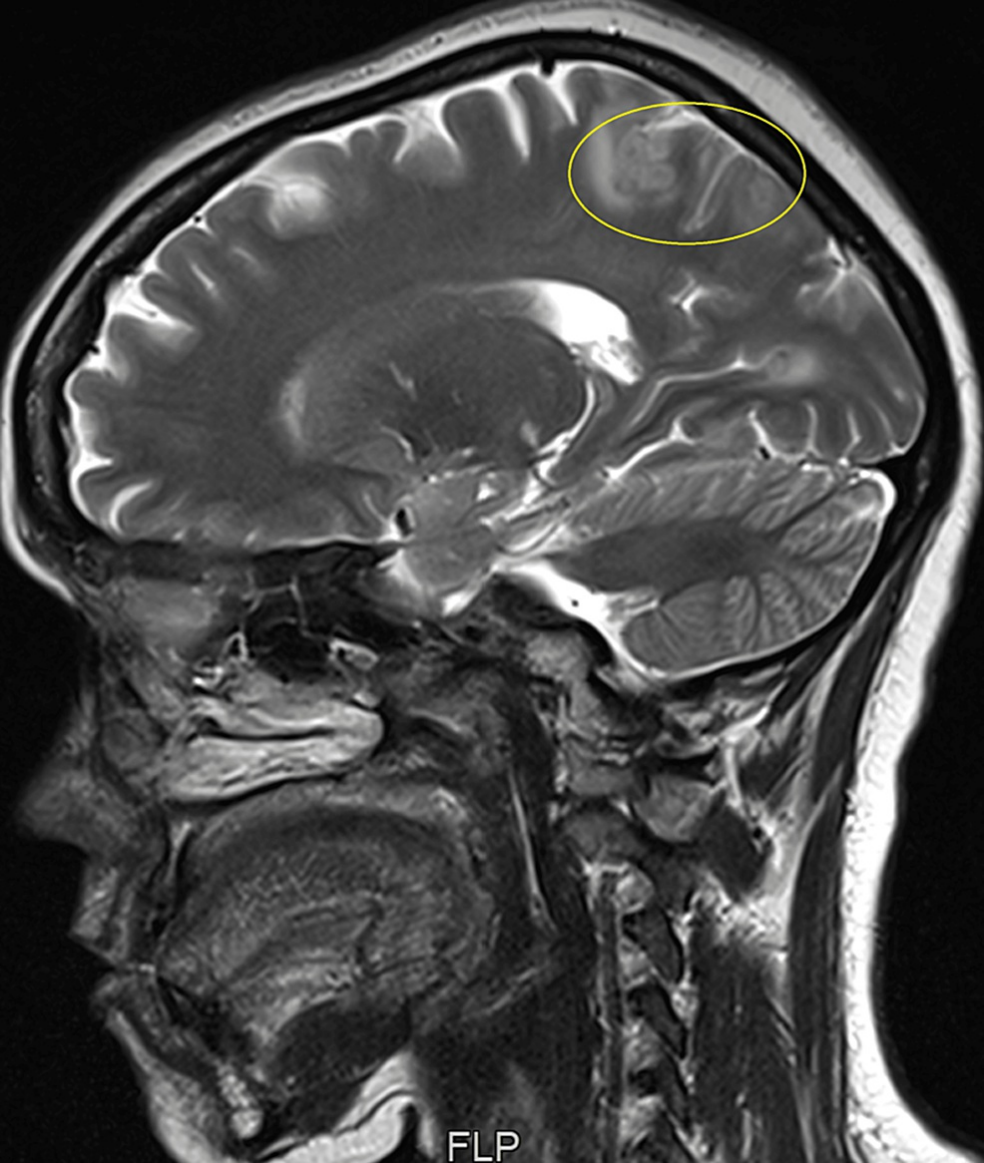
MRI brain sagittal section – multiple choroidal tubercles near corpus callosum
